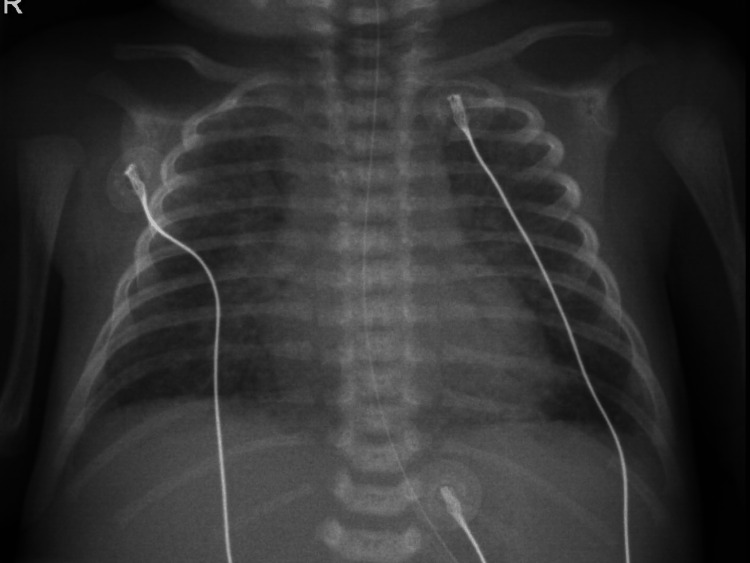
Congenital pneumonia. Chest radiograph, anteroposterior incidence, showing bilateral and diffuse interstitial pulmonary infiltrates, with air bronchogram, and partially sparing the left lung base, with no signs of pleural effusion or pneumothorax.
